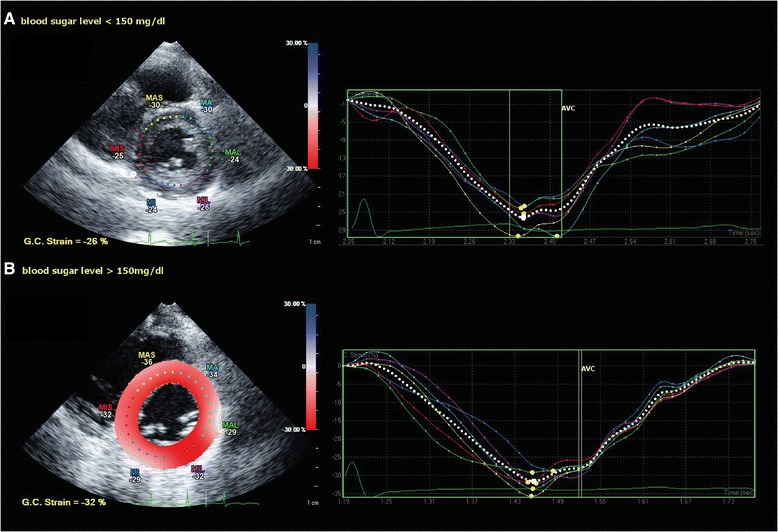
Speckle tracking echocardiography in the parasternal short axis view at level of the papillary muscles. a peak systolic global LV circumferential strain in a pediatric patient with type 1 diabetes mellitus and real time serum glucose level < 150 mg/dL. b peak systolic global LV circumferential strain in a pediatric patient with type 1 diabetes mellitus and real time serum glucose level > 150 mg/dL. *Note the increased (more negative) peak LV systolic global circumferential strain in the diabetic patient with the higher blood sugar level (*
